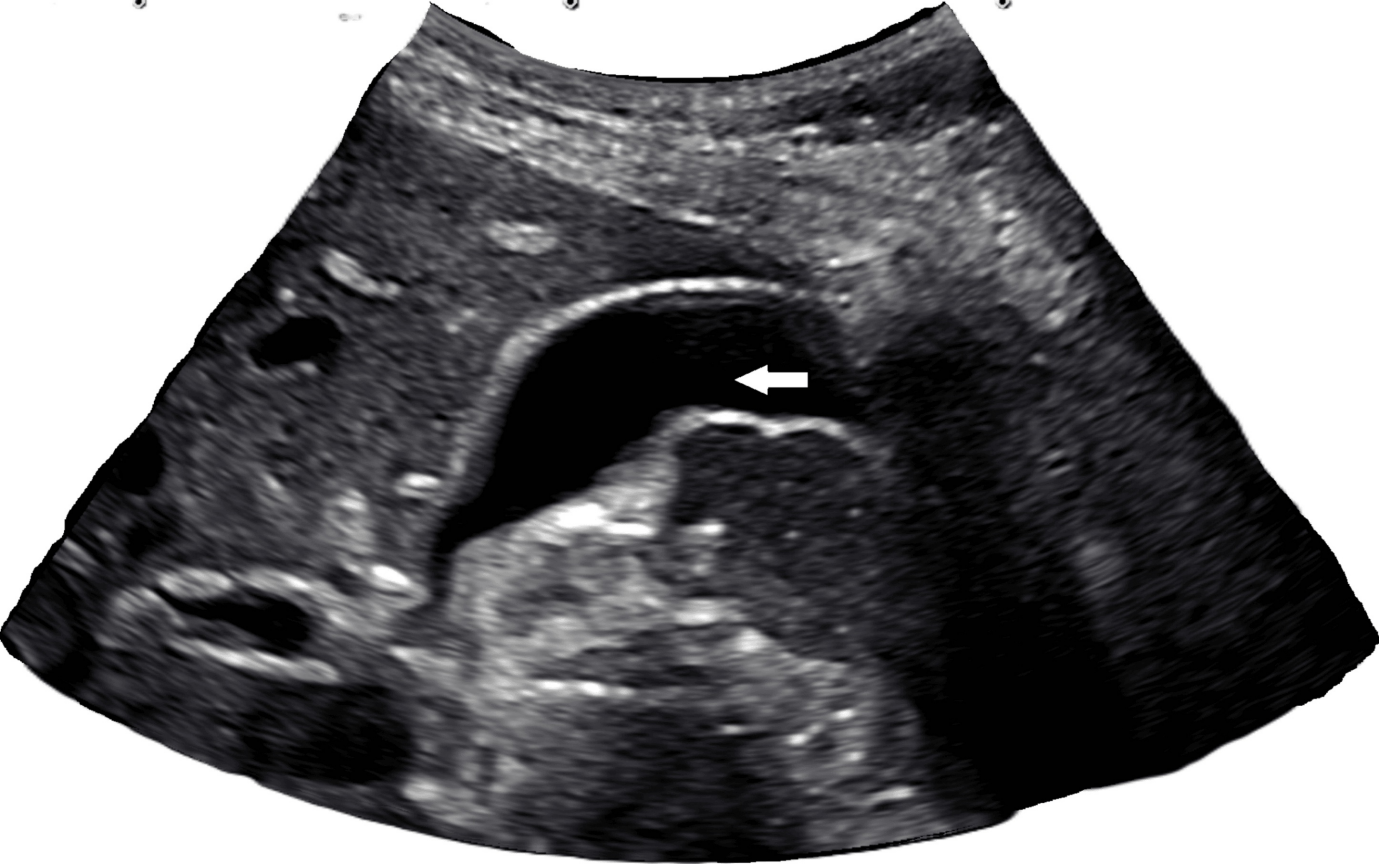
Right upper quadrant abdomen ultrasound of the gallbladder The right upper quadrant abdomen ultrasound of the gallbladder shows a normal gallbladder (arrow) without stones or dilatation.
